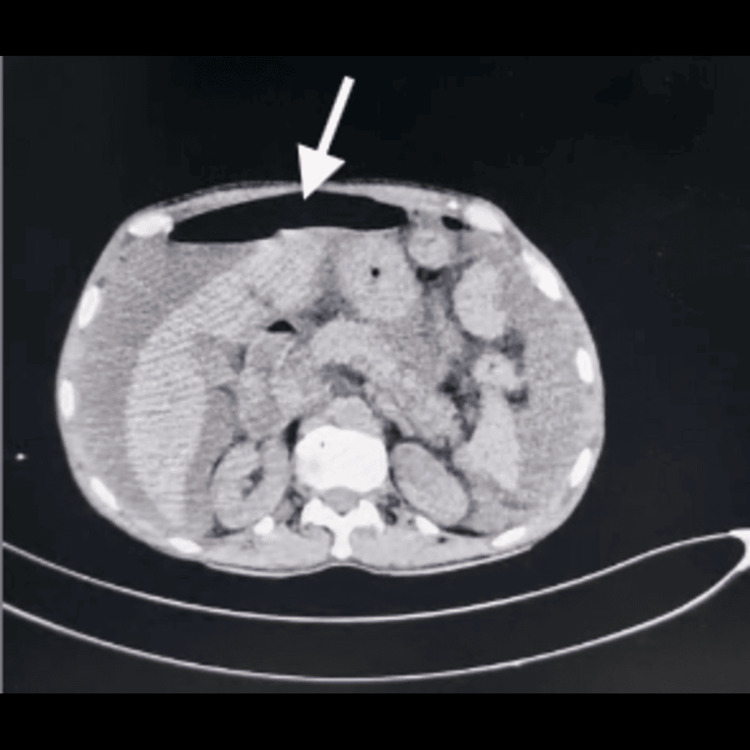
Non-contrast computed tomography of the abdomen demonstrated mild to moderate pneumoperitoneum (white arrow).
